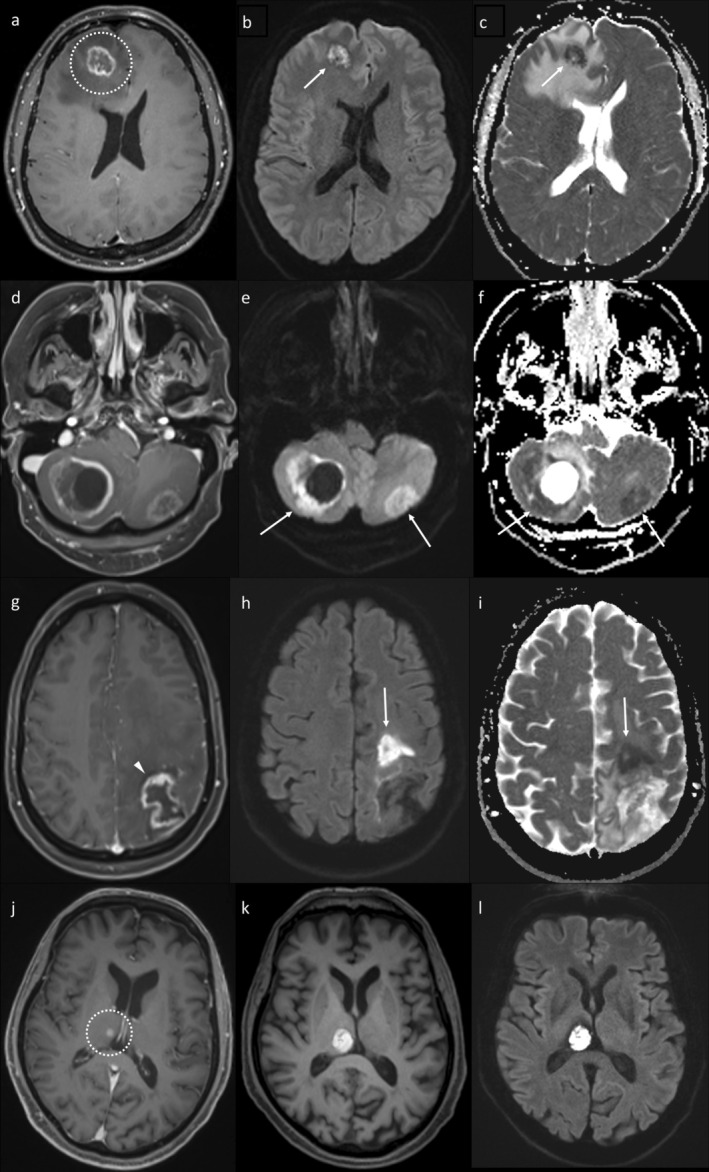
Different examples of the value of DWI in diagnosing RN and potential pitfalls. The top row (a–c) is an example of RN (dotted circle) demonstrating diffusion restriction (arrows) in the central non‐enhancing portion, with high signal on DWI (b) and low signal on the ADC map (c). The second row (d–f) demonstrates diffusion restriction in two cerebellar lesions in a patient with small cell lung cancer. Importantly, while there is some central diffusion restriction (high DWI signal, e, and low ADC signal, f), especially in the left cerebellar lesion, there is also diffusion restriction along the enhancing periphery; true tumour progression was confirmed at surgical resection of both lesions. The third row (g–i) shows an example of RN on post‐contrast T1WI (g) treated with bevacizumab, resulting in an area of presumed coagulative necrosis on subsequent imaging (presumably occurring within an area of less severe radiation injury), with high DWI signal (h) and low ADC (i). Other than for a decrease in the enhancement related to bevacizumab, the original lesion remained stable, confirming RN. The fourth row (j–l) demonstrates a metastasis (j, dotted circle), which developed haemorrhage, shown as high signal on pre‐contrast T1WI (k) and DWI (l).
